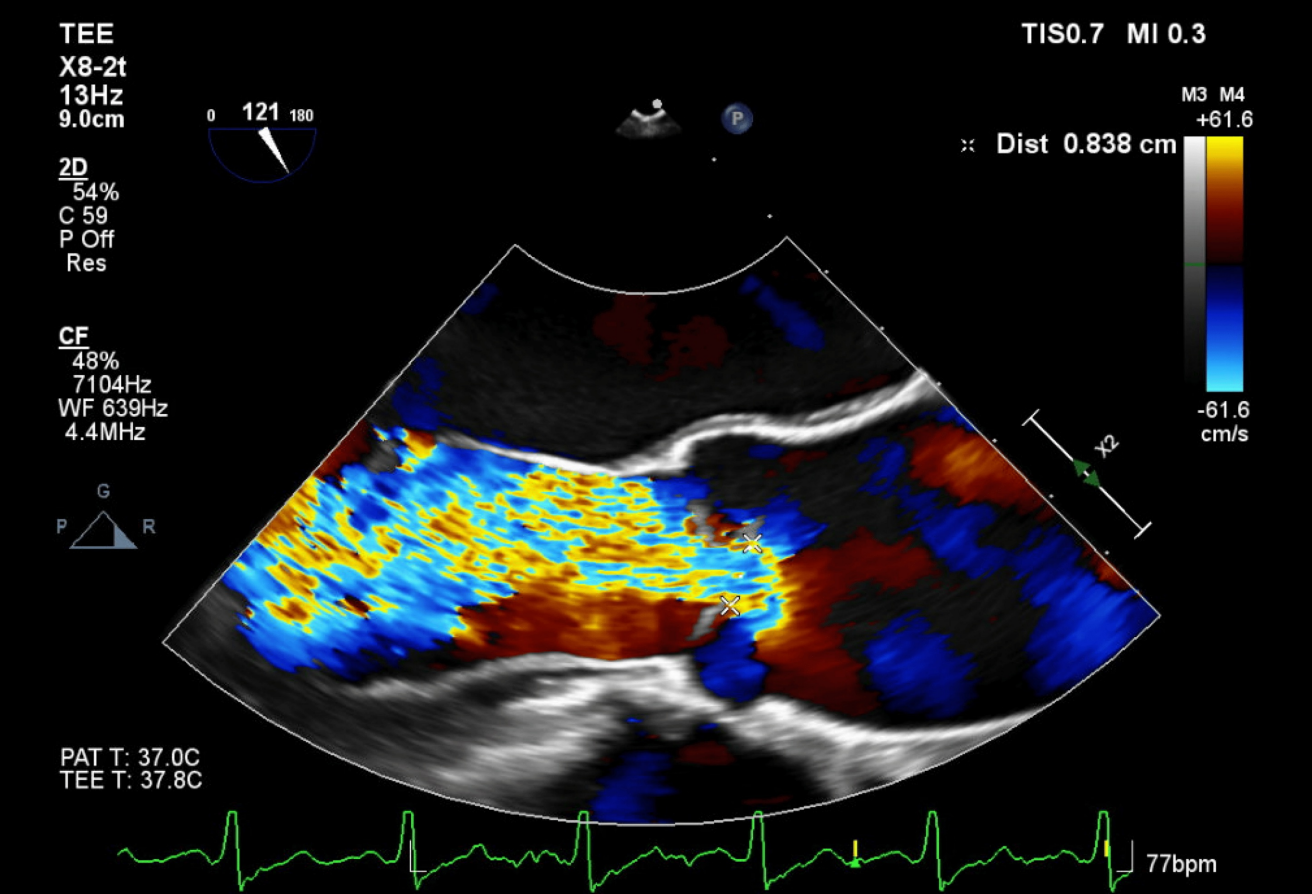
Transesophageal echocardiogram (TEE) demonstrating severe aortic regurgitation with a vena contracta of 0.83 cm.
